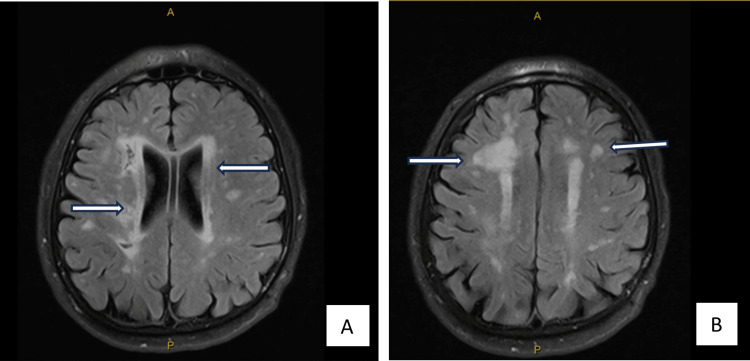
Brain MRI without contrast showing extensive periventricular and subcortical white matter disease, including external capsule (A; white arrows) and subcortical white matter of the temporal lobe (B; white arrows) MRI: magnetic resonance imaging
